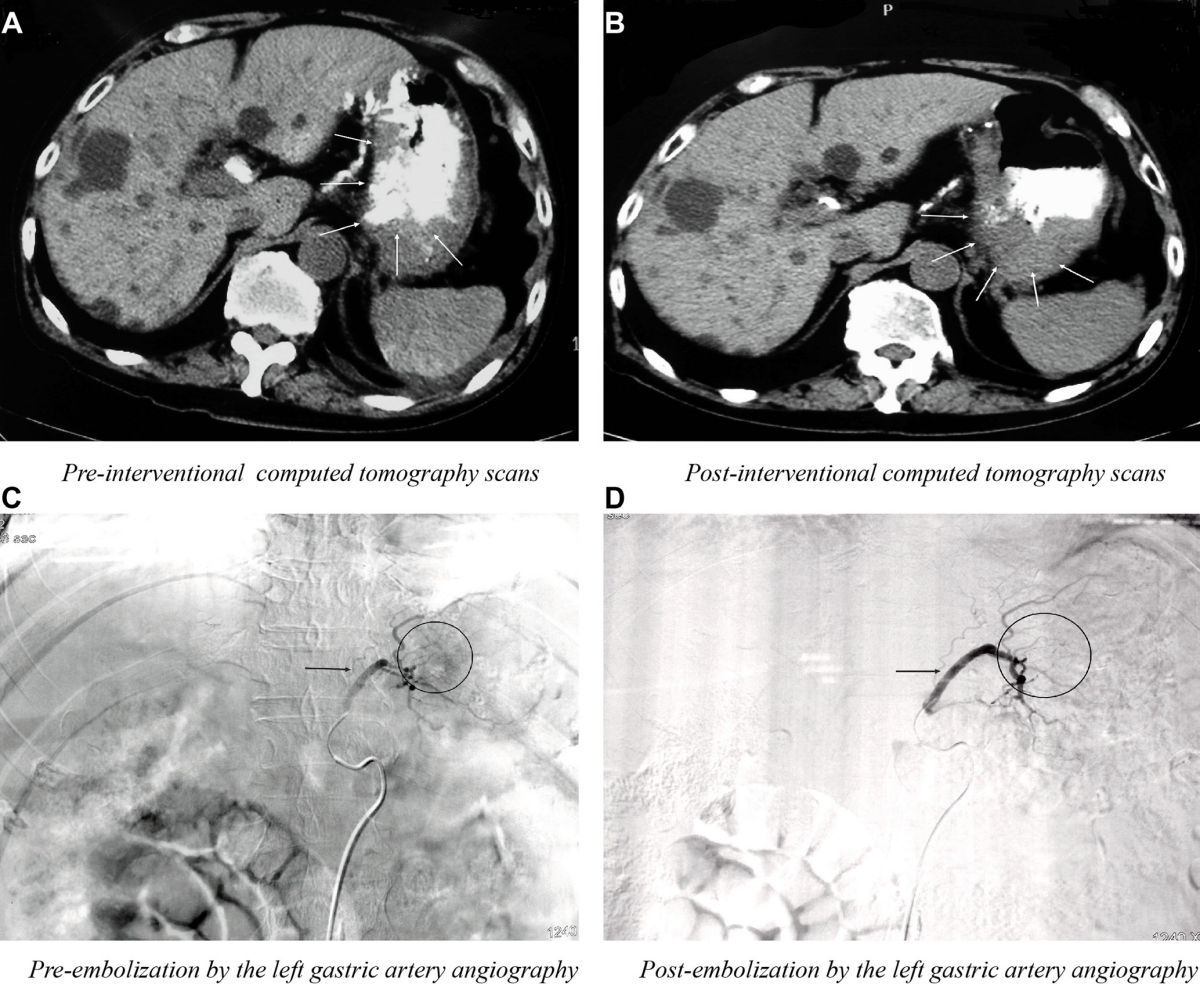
The results of pre-interventional (A) post-interventional (B) computed tomography scans, pre-embolization (C) and post-embolization (D) by the left gastric artery angiography.
